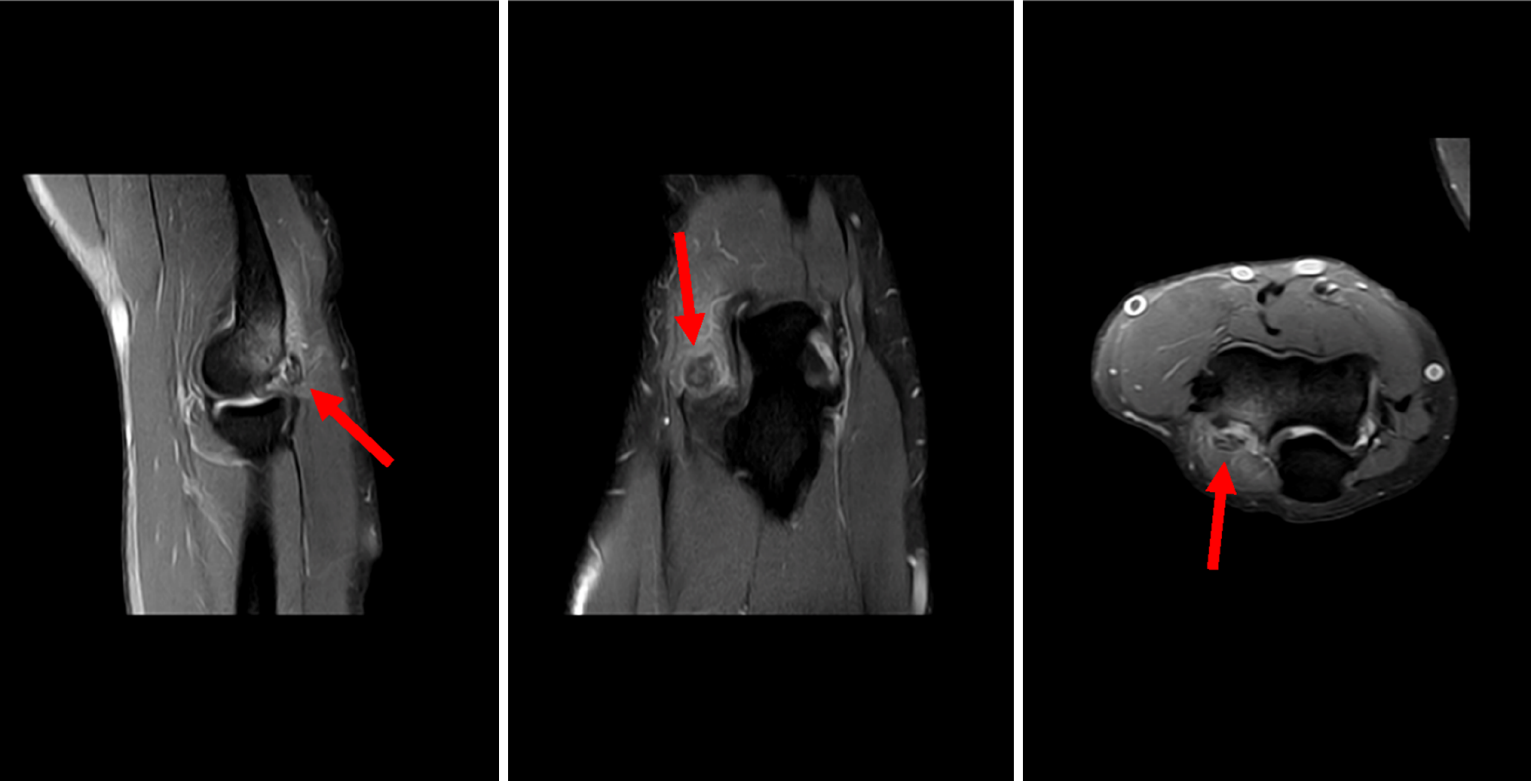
MR images of the right elbow of patient no. 8 at first presentation (the arrow marks the signal enhancement at the posterior aspect of the elbow)
